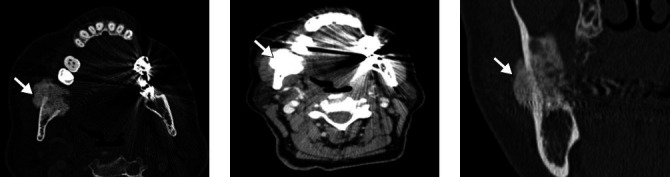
CT scans of a 57-year-old female patient, showing a lobulated exophytic
